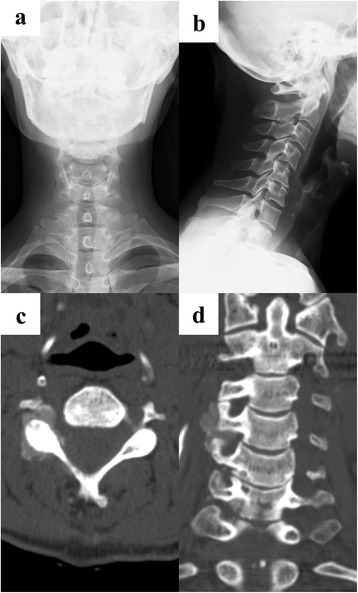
X-ray images show no remarkable change in anteroposterior view (a) and slight kyphosis in lateral view (b). Axial computed tomography scan image demonstrates lobulated and extraosseous calcified mass in the intervertebral foramen of fourth-fifth cervical spine (c). Coronal computed tomography scan image also demonstrates lobulated and extraosseous calcified mass at right side of spine (d)
